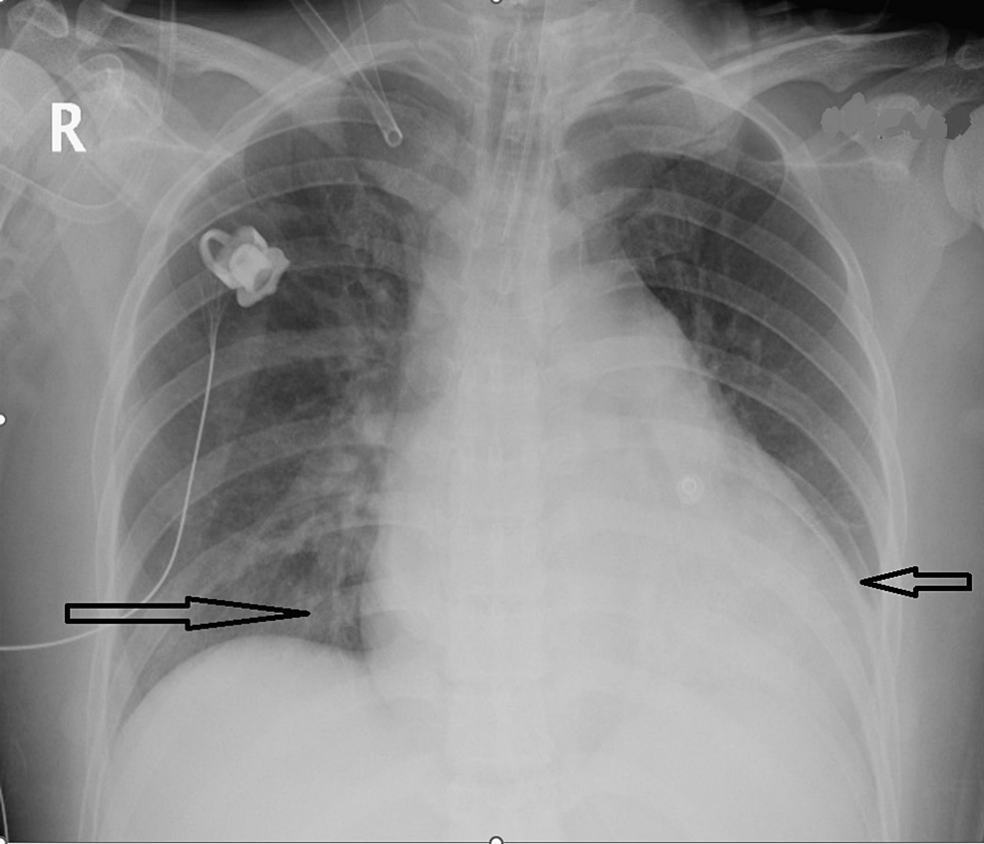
Chest x-ray showing cardiomegaly and patchy consolidations bilaterally in the mid and lower lung fields
